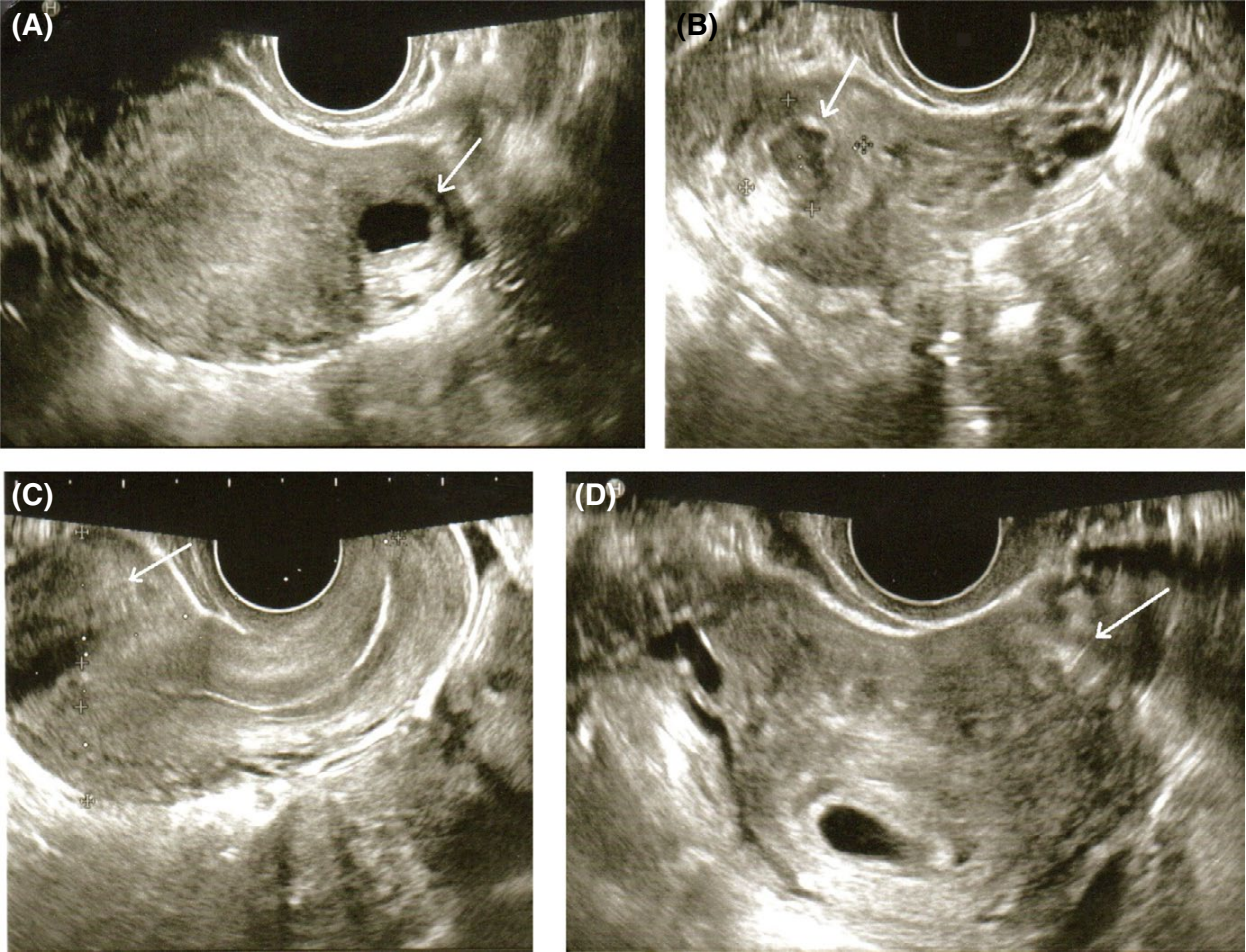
Vaginal pelvic ultrasound: A, a pre‐sclerotherapy cross‐sectional view of the ACUM near the left uterine horn. The white arrow indicates the ACUM or its former site. B, a parasagittal view during sclerotherapy of the ACUM, after ethanol injection. The white arrow indicates the ACUM or its former site. C, a post‐sclerotherapy parasagittal view at 1 mo (disappearance of the ACUM). The white arrow indicates the ACUM or its former site. D, a cross‐sectional view at the start of pregnancy (6 wk of amenorrhea), 3 mo after sclerotherapy (disappearance of the ACUM). The white arrow indicates the ACUM or its former site
